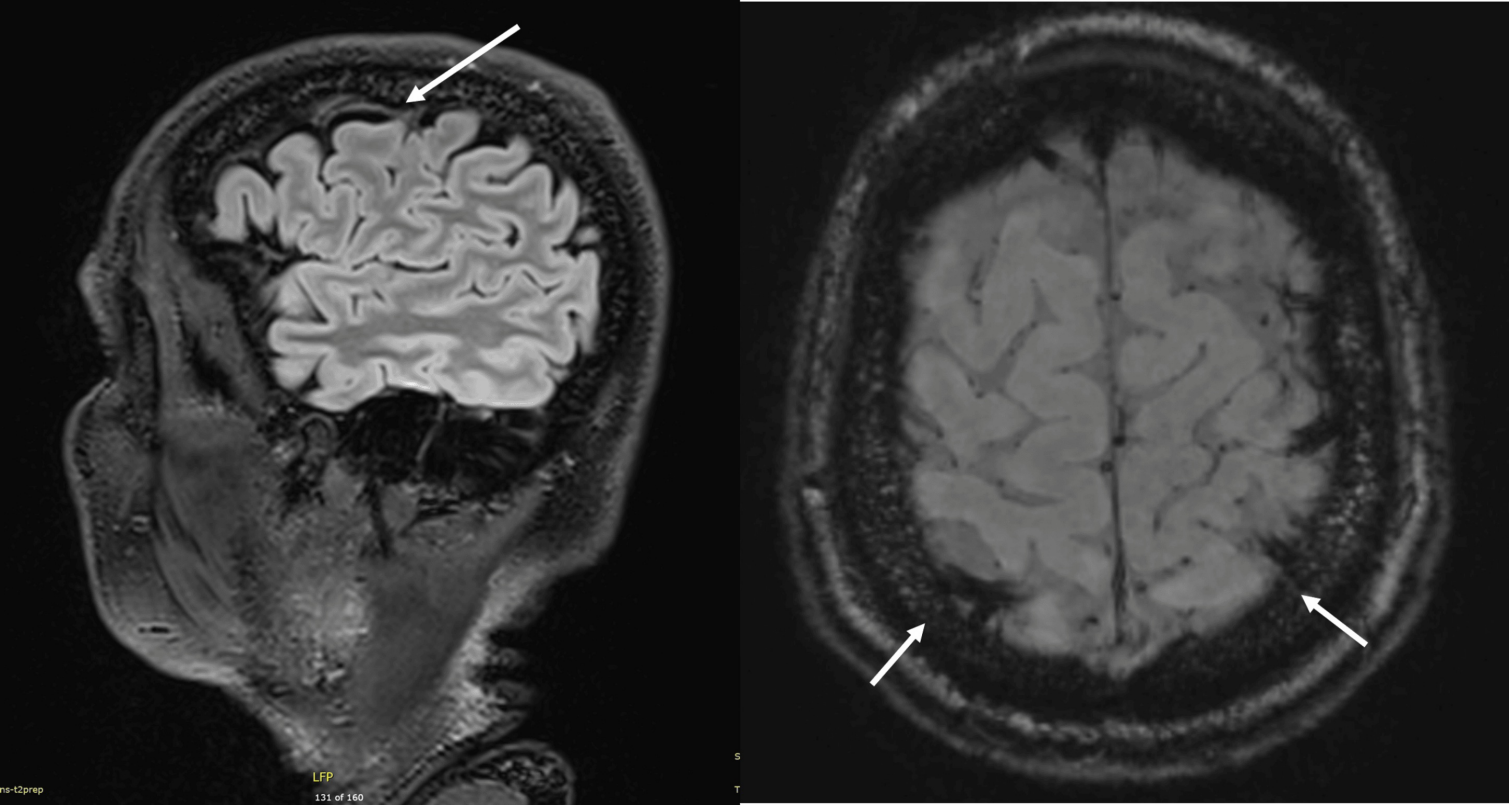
A follow-up MRI of the brain (19/09/24) image showed a reduced T2/fluid-attenuated inversion recovery (FLAIR) signal indicated on the image on the left. Magnetic resonance susceptibility-weighted imaging again showed abnormally vessel thickening corresponding to thrombi.
